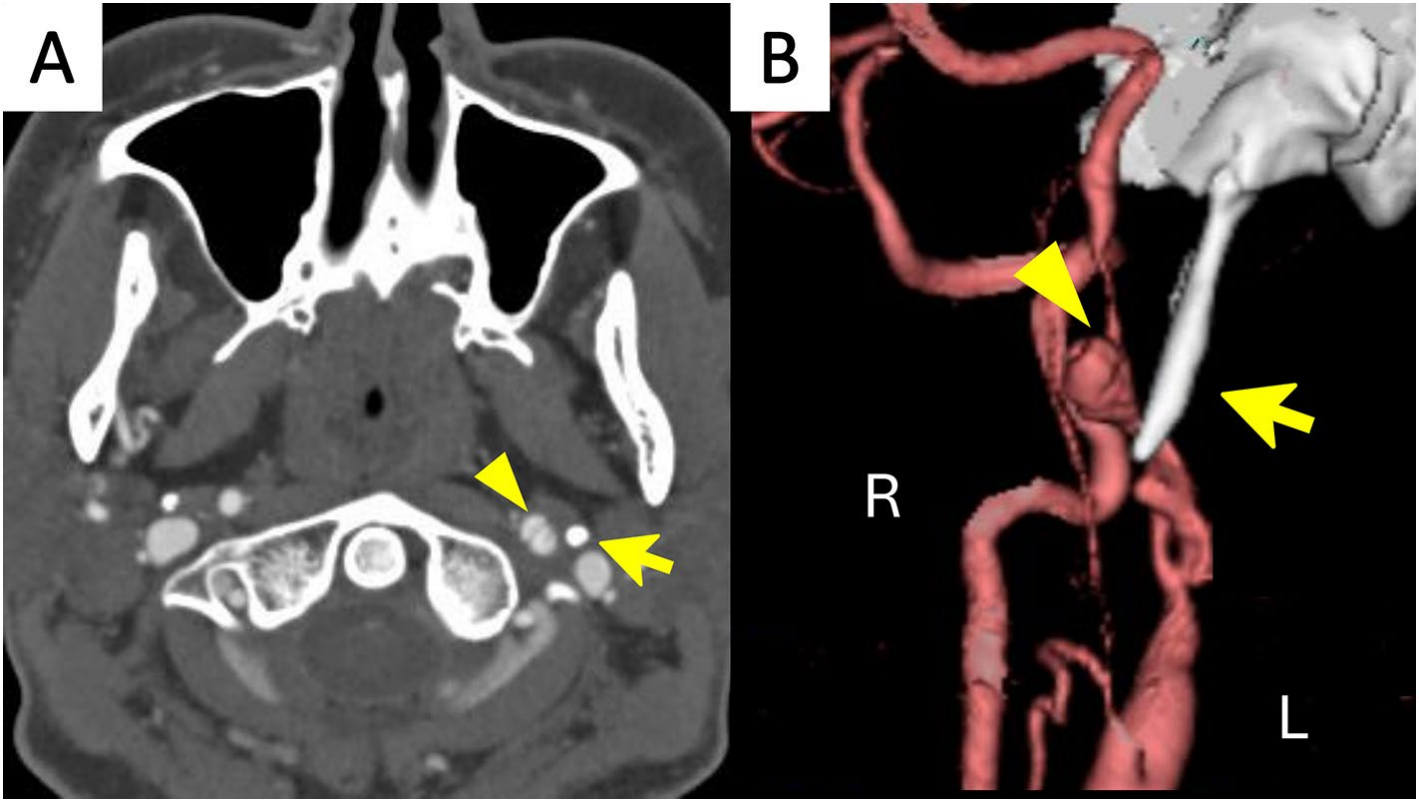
Computed tomography angiography (CTA) performed on the day of admission showing dissection of the left internal carotid artery with intimal flap (arrowhead) and an elongated styloid process (arrow). Original image (A) and 3D reconstructed image (B)
